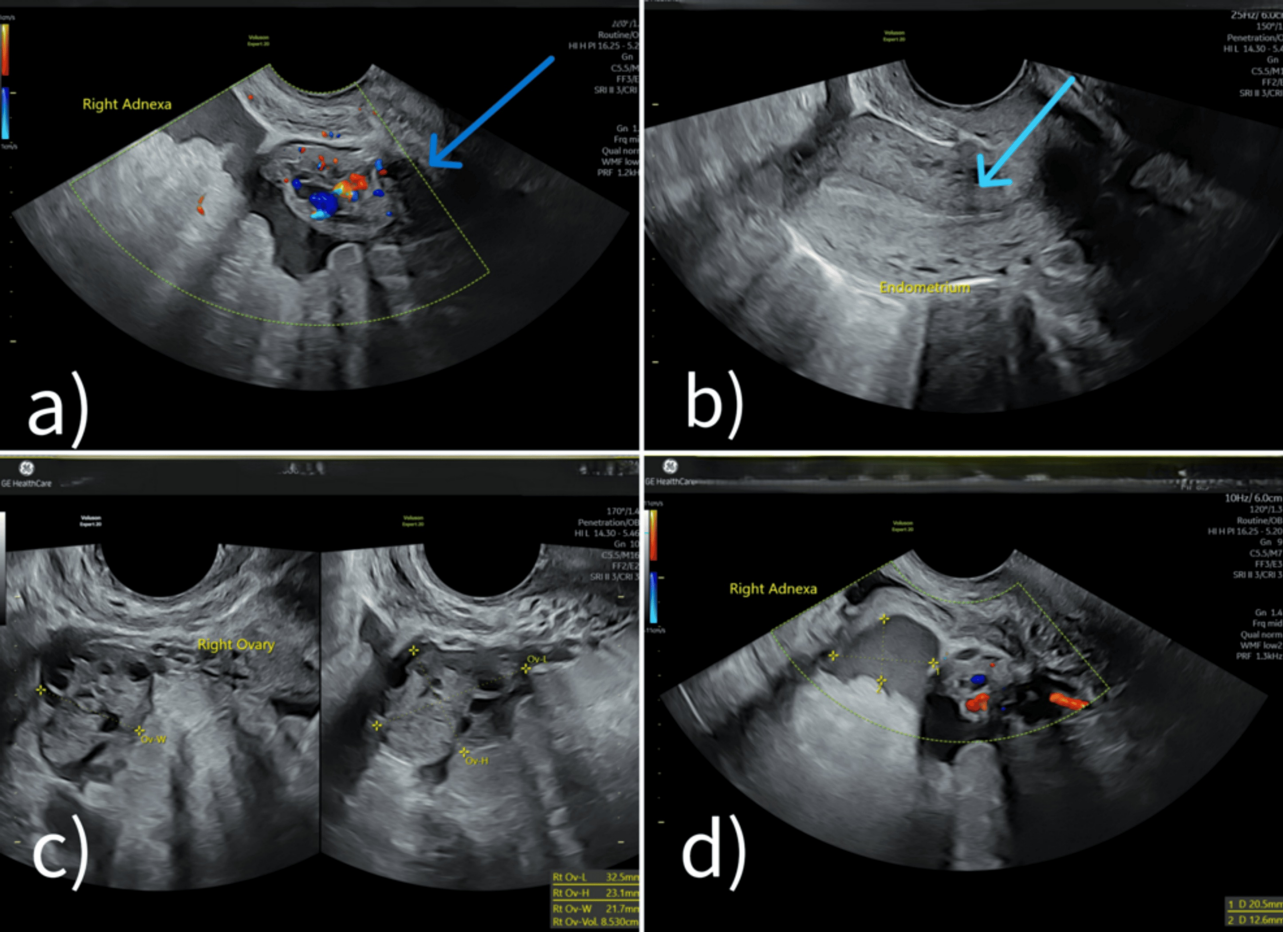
Transvaginal ultrasound images (a) Right adnexa with color Doppler showing a heterogeneous adnexal mass with internal vascularity. (b) Endometrium appears empty, without evidence of an intrauterine gestational sac. (c) Right ovary with the adnexal mass. (d) Right adnexa with color Doppler showing a well-defined, rounded mass with vascularity
